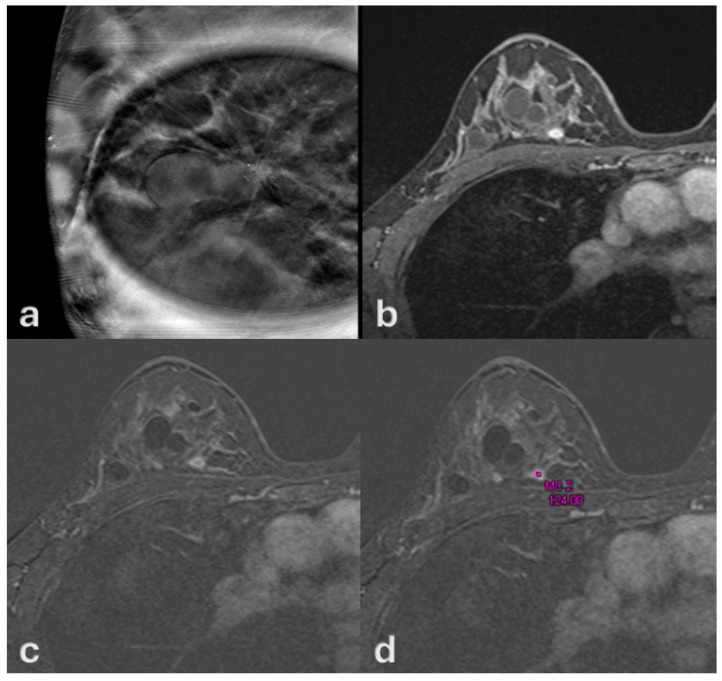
A 53-year-old woman with no history of oral contraceptive use. (a) Digital mammography, craniocaudal view, demonstrates architectural distortion in the right breast associated with clustered microcalcifications. (b–d) Contrast-enhanced MRI depicts a 6 mm lesion with gradual enhancement without washout, consistent with a type II kinetic curve. Surgical pathology confirmed high-grade ductal carcinoma in situ (DCIS).
